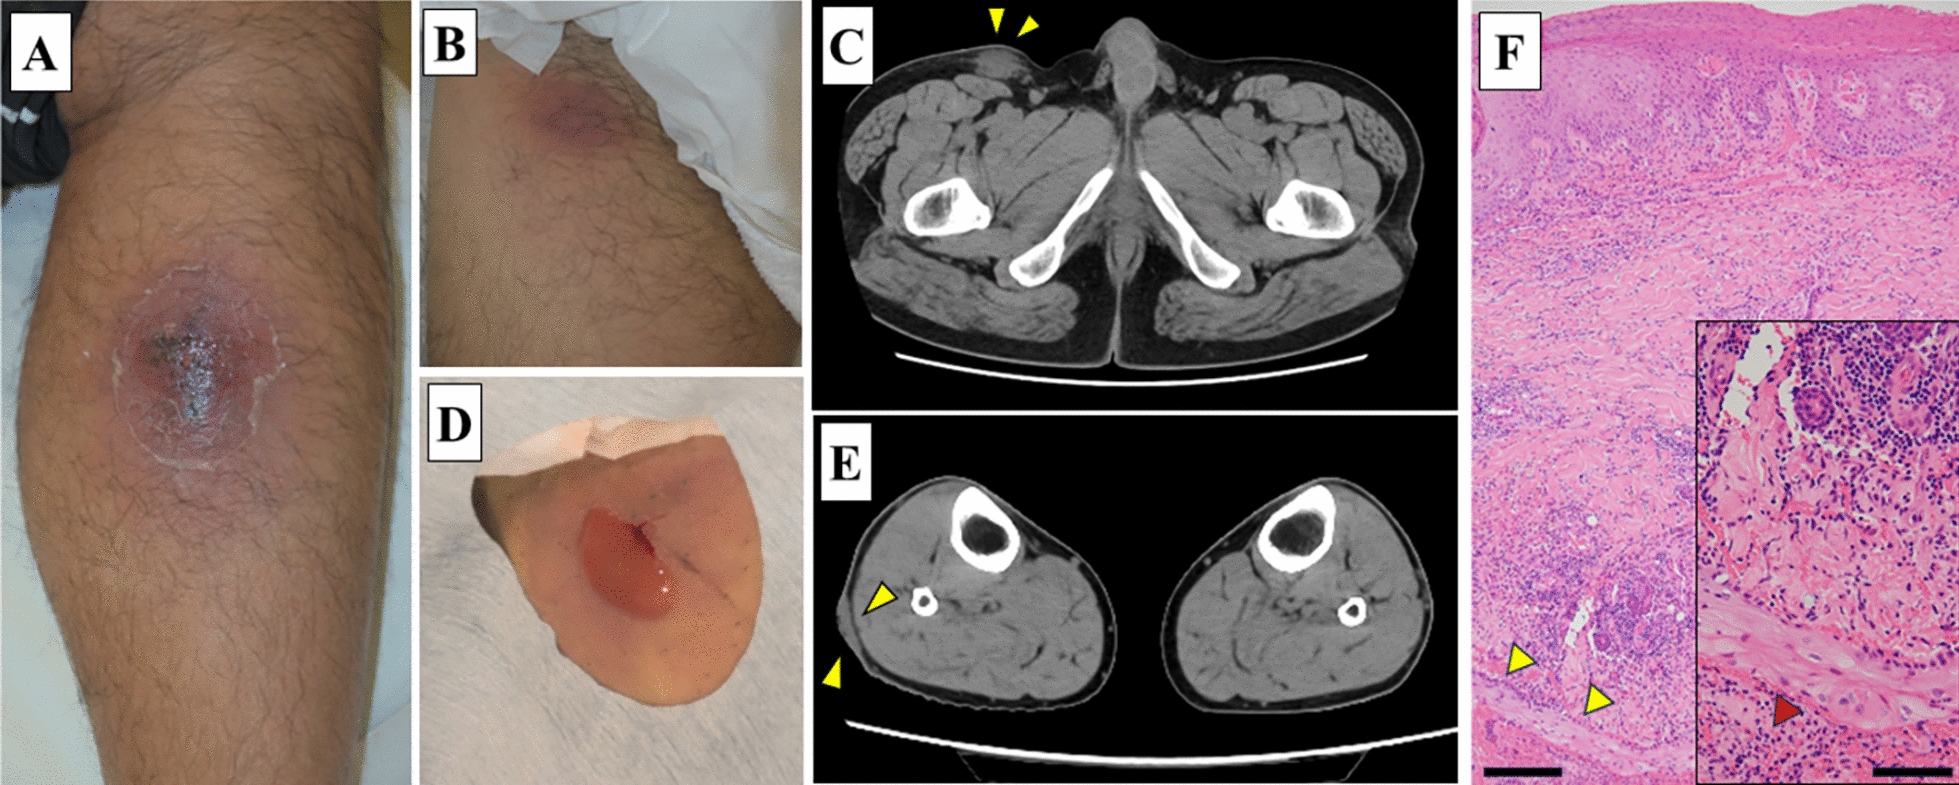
Image of findings. A 7 × 6 cm cutaneous abscess with a reddish surface and exudation. B Reddish and swelling lesion in the right inguinal region. C Computed tomography showing lymphadenitis in the right groin (the arrowheads). D Red and cloudy pus discharge through incision at the right inguinal region. E Computed tomography showing a cutaneous infection in the right posterior lower extremity (the arrowheads). F Hyperkeratosis and parakeratosis are observed in the epidermis layer. Inflammation caused mainly by lymphocytes is observed in the dermis layer, and fragments of stratified squamous epithelium without atypia are observed (the yellow arrowheads) (bar 200 μm). Neutrophil infiltration is observed under the squamous epithelium in inset (the red arrowhead) (bar 50 μm)
